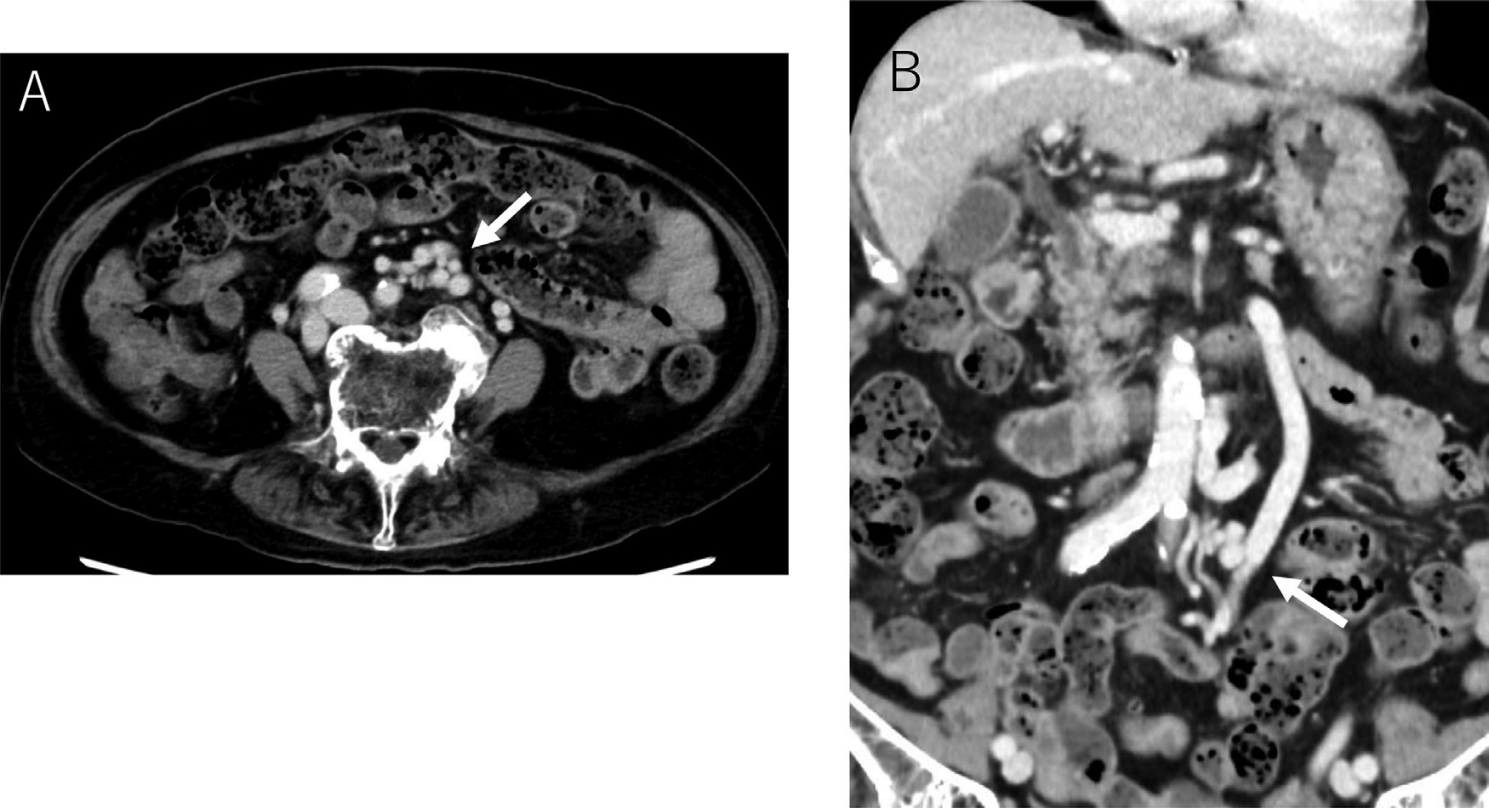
Contrast-enhanced CT before treatment. (A) Contrast-enhanced CT before treatment shows shunt vessels (white arrow). (B) Coronal view of contrast-enhanced CT before treatment shows dilated inferior mesenteric vein (IMV) (white arrow).
